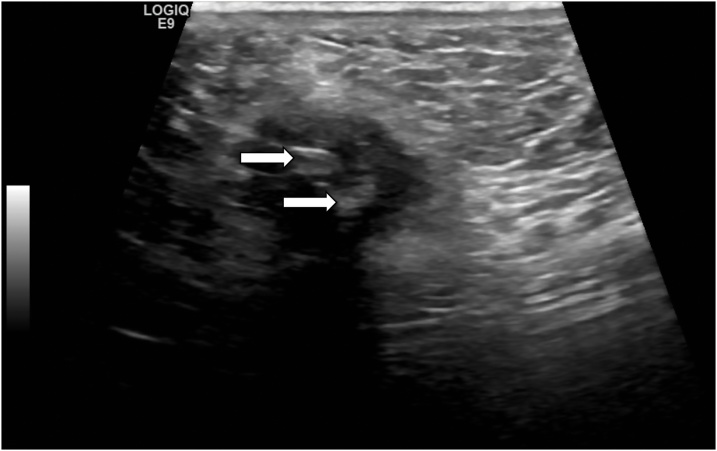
Ultrasound showing two echogenic masses representing gallstones.
